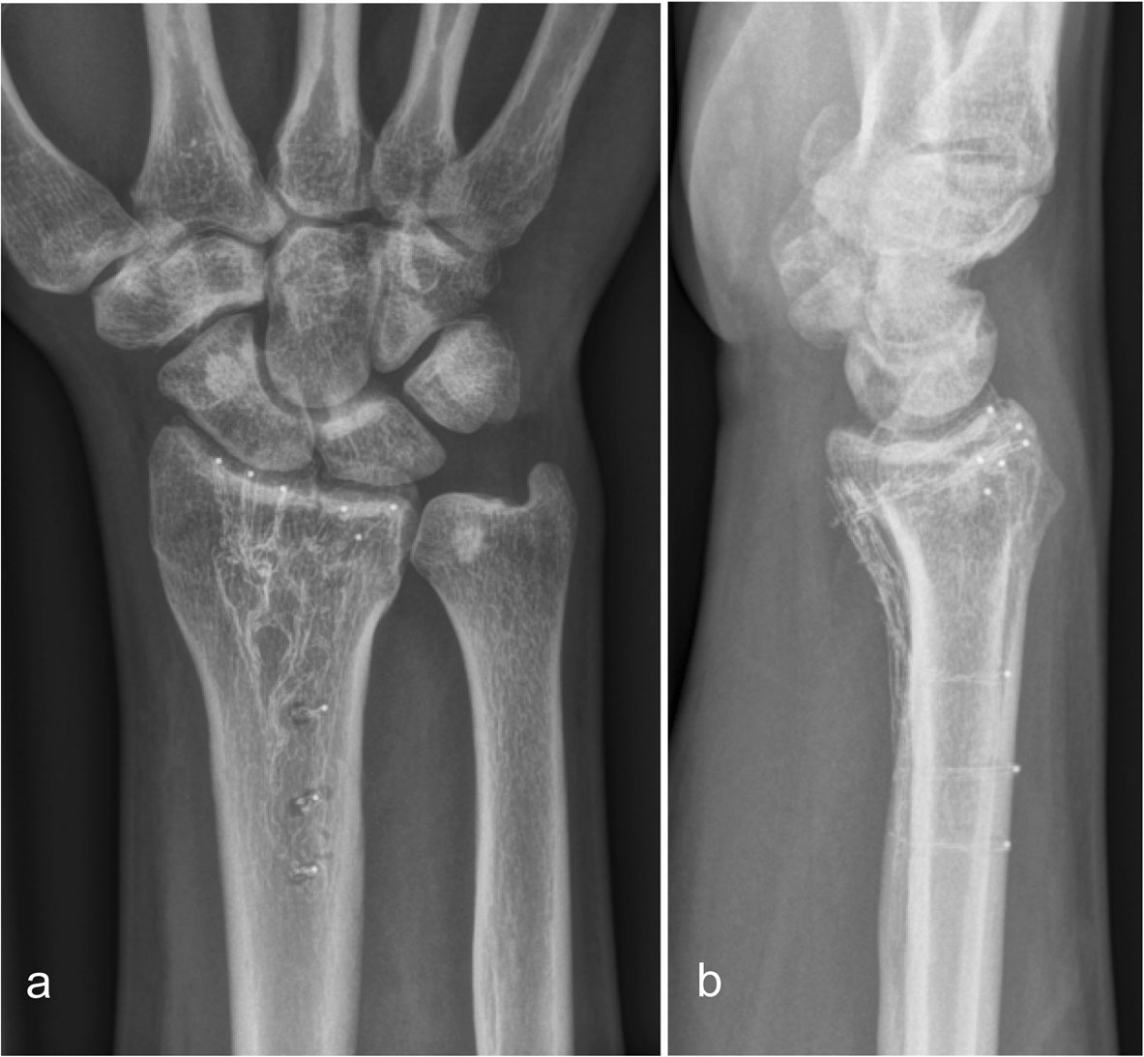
Distal radius fracture treated with a 2.7 mm Carbon/PEEK plate. X-ray 12 weeks after surgery, ap (a) and lat view (b)
